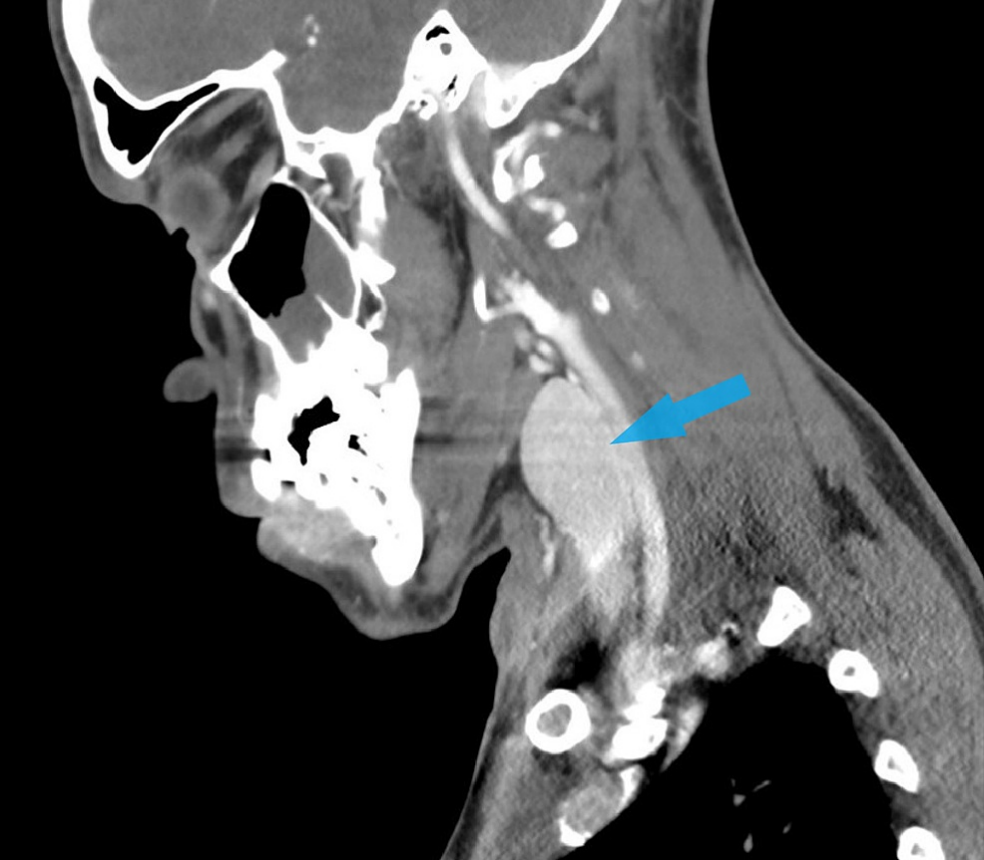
CT soft tissue neck with IV contrast showing HS abscess in the right neck. HS: hidradenitis suppurativa
